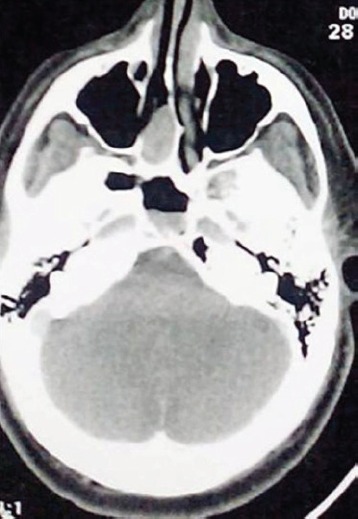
CT showing a soft tissue mass from the right nasal cavity extending to the posterior ethmoid air cells. Inflammatory changes are present to the right sphenoid sinus.
